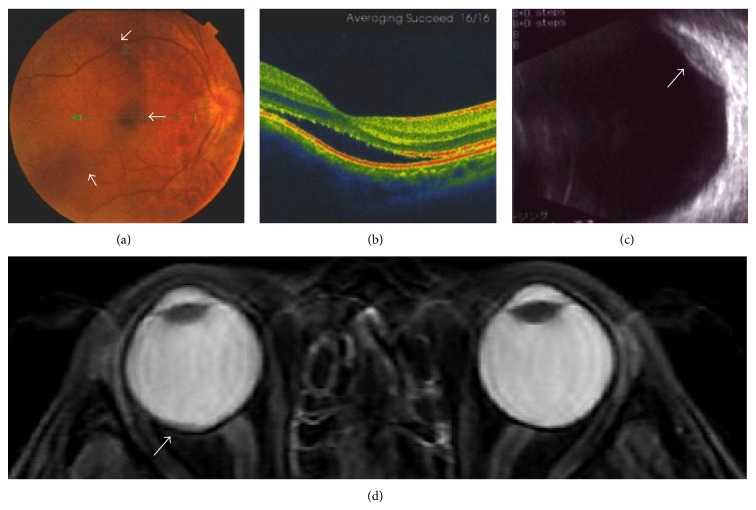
An elevated choroidal neoplasm. (a) Fundoscopic findings of the right eye demonstrating choroidal metastasis (arrows). (b) Optical coherence tomographic findings showing subretinal fluid in the inferior aspect of the lesion compromising the fovea. ((c) and (d)) Ultrasound and MRI T2-weighted images demonstrating an elevated choroidal mass (arrow).
